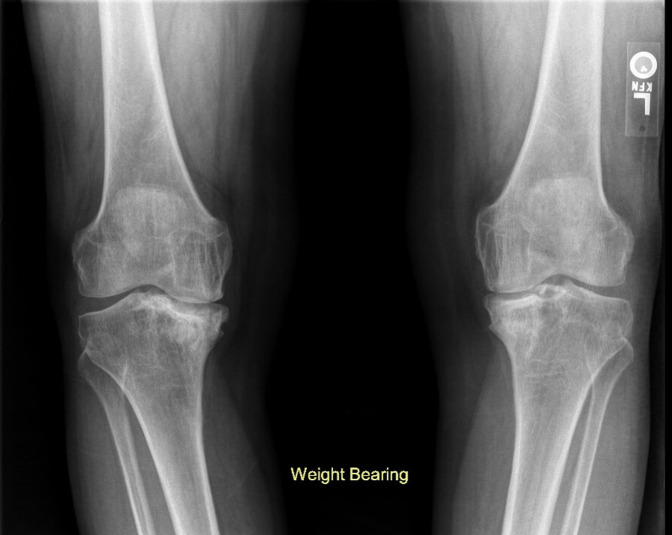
Follow-up anterior-posterior weight-bearing plain radiographs of both knees demonstrating progressive collapse of the medial tibial plateau with varus malalignment and secondary osteoarthritis bilaterally more severe on the right.
